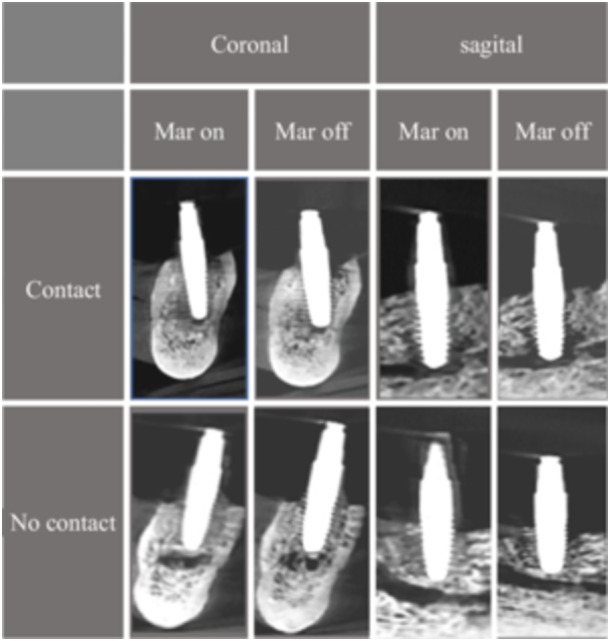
Tangential and cross‐sectional CBCT views of a zirconia implant with and without contact with the IAC in MAR Off and On conditions.
